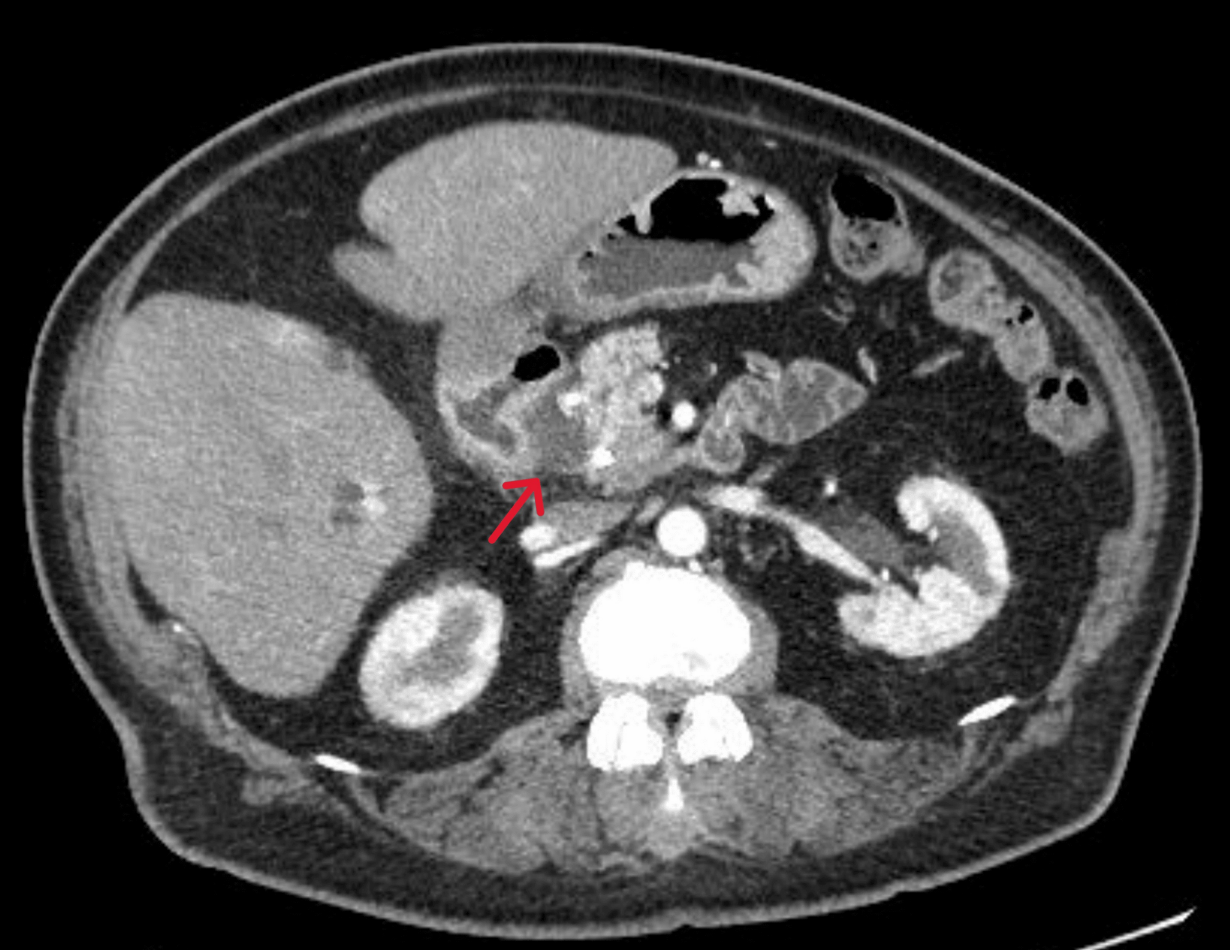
Abdomen CT with contrast showing the presence of a soft tissue mass (red arrow).
